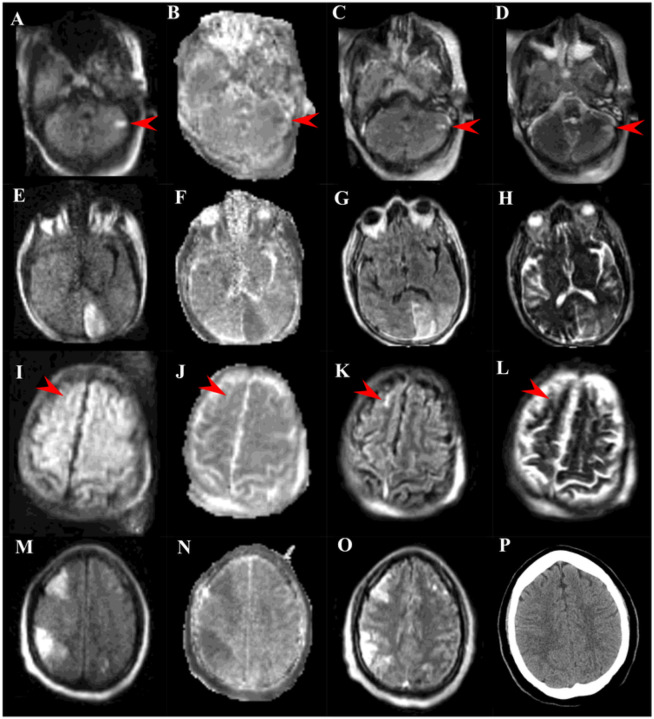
Ultra-low-field portable MRI (ULF-pMRI) images and head CT image in patients with extracorporeal membrane oxygenation (ECMO) support. Images A, E, I, M are diffusion-weighted imaging (DWI) MRI, images B, F, J, N are apparent diffusion coefficient MRI, images C, G, K, O are fluid-attenuated inversion recovery (FLAIR) MRI, images D, H, L are T2-weighted MRI, and image P is a CT scan. Patient 1 (Images A–D) demonstrated small ischemic stroke in the left cerebellum denoted by red arrows. Patient 2 (E–H) demonstrated moderate ischemic stroke in the left occipital lobe. Patient 3 (I–L) had an intracranial hemorrhage in the right superior frontal sulcus (red arrow). Patient 4 (M-O) demonstrated multifocal infarcts in the bilateral superior frontal and parietal lobes on MRI images and had a normal CT scan. Entire series of MRI sequences and images for these patients are available as the link to Digital Imaging and Communications in Medicine (DICOM) in the Hyperfine, Inc. Imaging Viewer.
